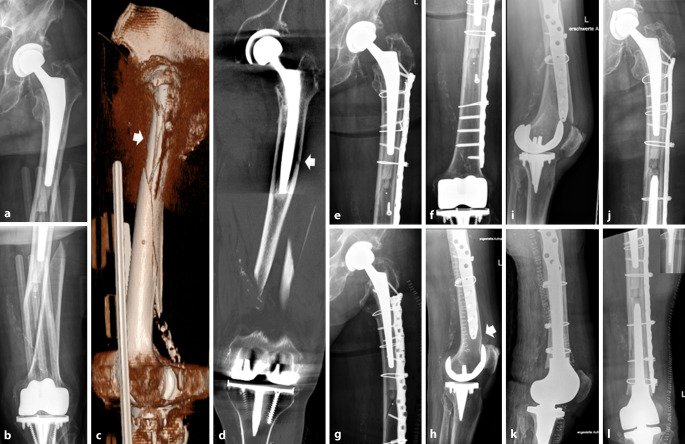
Fallbeispiel: 90 Jahre, männlich. a, b Konventionell radiologisch zeigt sich eine langstreckige interprothetische Spiralfraktur bei nicht gelockerter teilzementierter Hüfttotalendoprothese und festsitzendem bikondylärem Oberflächenersatz. c, d In der erweiterten Schnittbildgebung mittels CT zeigt sich eine mehrfragmentäre Frakturmorphologie mit Ausläufern nach proximal bis unterhalb des Trochanter-major-Massivs (*Pfeil*). e–h Postoperative Kontrolle nach langstreckiger winkelstabiler Plattenosteosynthese (Fa. Zimmer, NCB proximal femur plate) des Femurschaftes. Ganz distal zeigt sich in der lateralen Projektion ein fissuraler Frakturausläufer der ventralen Femurkortikalis (*Pfeil*). i Erneute notfallmäßige Vorstellung 6 Wochen nach der osteosynthetischen Versorgung bei stattgehabtem Sturzereignis aus dem Pflegebett. Konventionell radiologisch zeigt sich eine dorsale Abscherfraktur des distalen Femurs. j–l Postoperative Kontrolle nach Revisionsoperation mit Resektion des distalen Femurs und Re-Osteosynthese mit Rekonstruktion der femoralen Schaftkonfiguration mittels Cable-Ready-Cerclagen über die einliegende Plattenosteosynthese sowie Implantation eines modularen zementierten distalen Femurersatzes mit gekoppelter Kniegelenkendoprothese (Fa. Link, Typ Mega C). Der femorale Abstand der Schaftspitzen beträgt weniger als 11 cm, allerdings stabilisiert die langstreckige Plattenosteosynthese protektiv. Postoperativ wurde die schmerzadaptierte Belastung freigegeben
